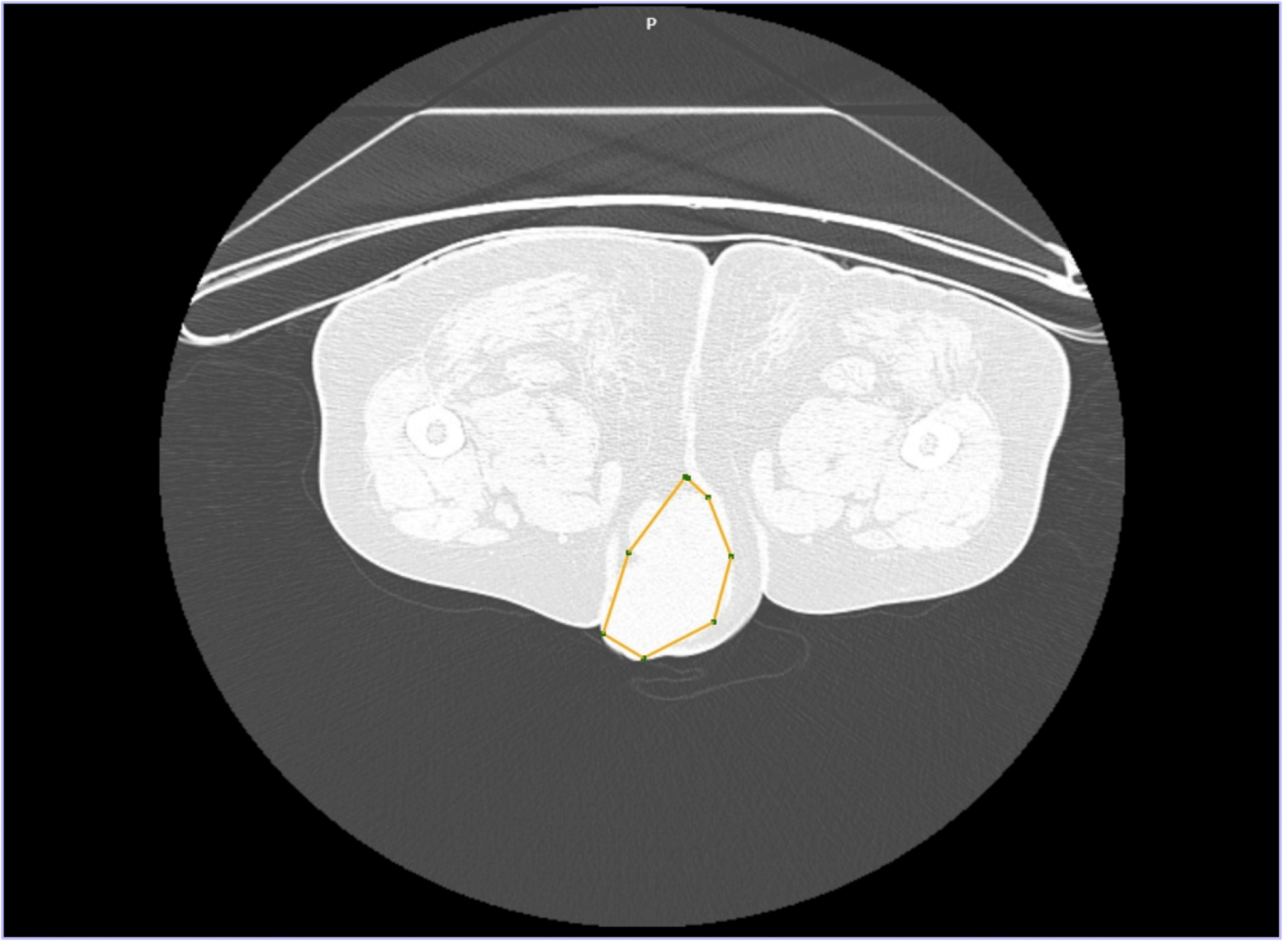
CECT showing a large ill-defined lobulated soft tissue density lesion noted arising from the vulva measuring 6×7.9×8.5 cm CECT: contrast-enhanced computed tomography
